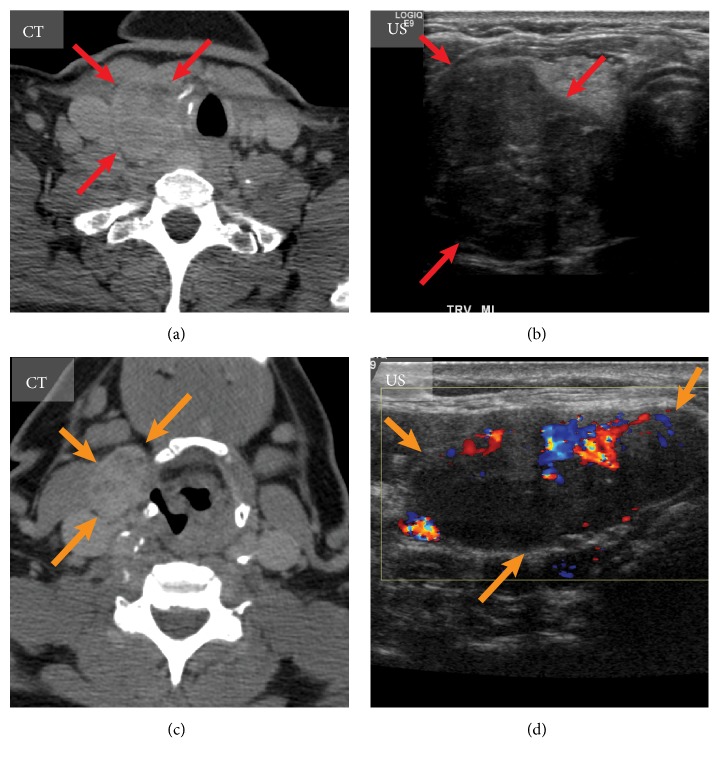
Imaging of the medullary thyroid carcinoma with metastasis to lymph node. Medullary thyroid carcinoma of the right thyroid lobe (red arrows) visualized on axial computed tomography (CT) (a) and transverse ultrasound (US) scan (b). A large metastatic lymph node (orange arrows) is demonstrated on axial CT of the neck (c) and sagittal US (d).
